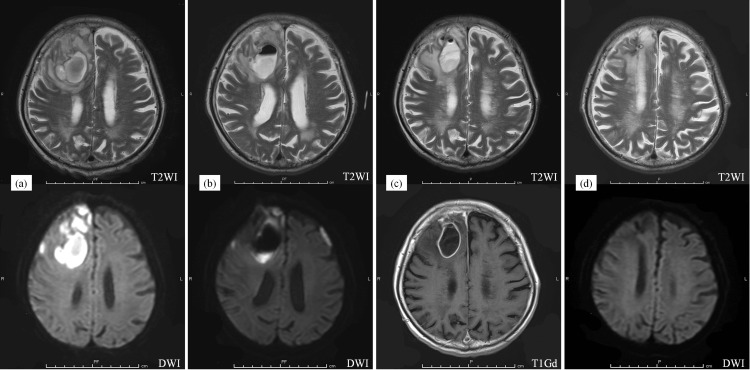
MRI scans obtained on different days after admission. (a) Day 4: a polycystic lesion in the right frontal lobe of the patient’s brain with high intensity on the T2-weighted image (T2WI) and diffusion-WI. (b) Day 11: shrinkage of the brain lesion. (c) Day 39: fluid re-accumulation in the lesion space after drainage. On the right side is a gadolinium-enhanced T1 weighted-image (T1Gd). (d) Day 193: shrinkage of lesion and non-recurrence of the abscess.
